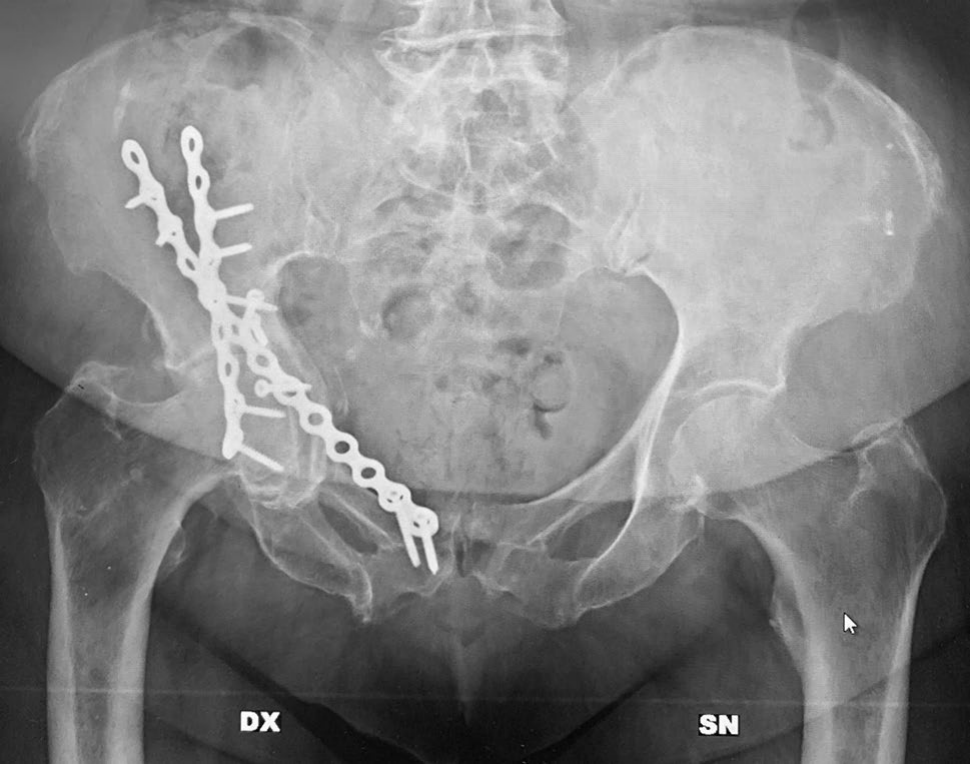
An anteroposterior X-ray in a 78-year-old lady. As opposite of the previous case, note the anatomical reduction of the quadrilateral plate that led to fracture healing without the further development of the osteoarthritis
